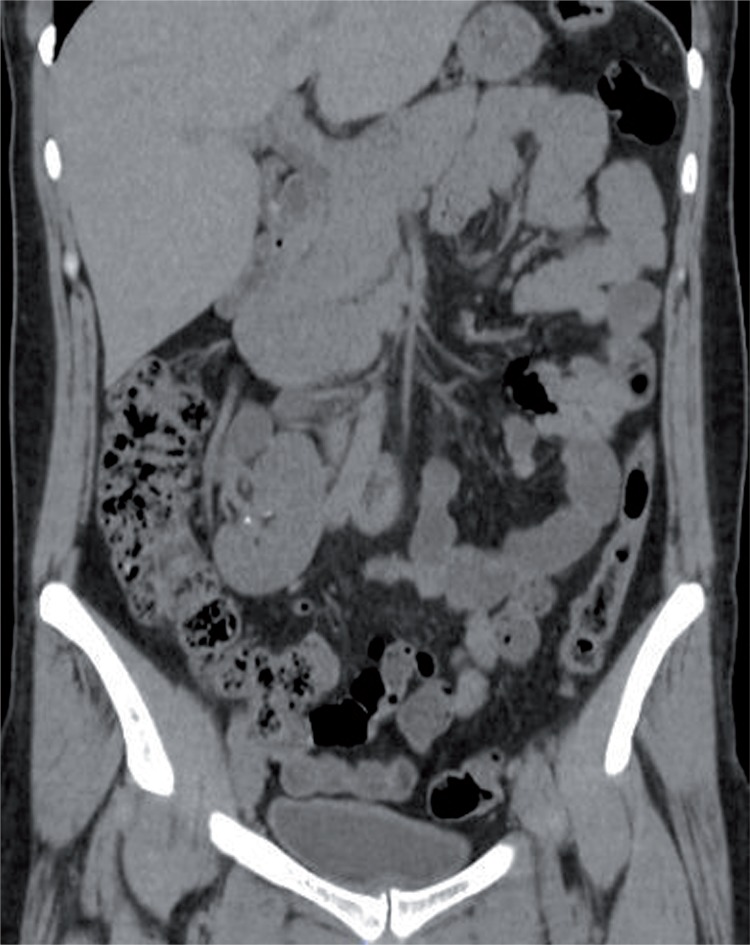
Computed tomography scan of supernumerary kidney with its own blood supply and collecting system.
